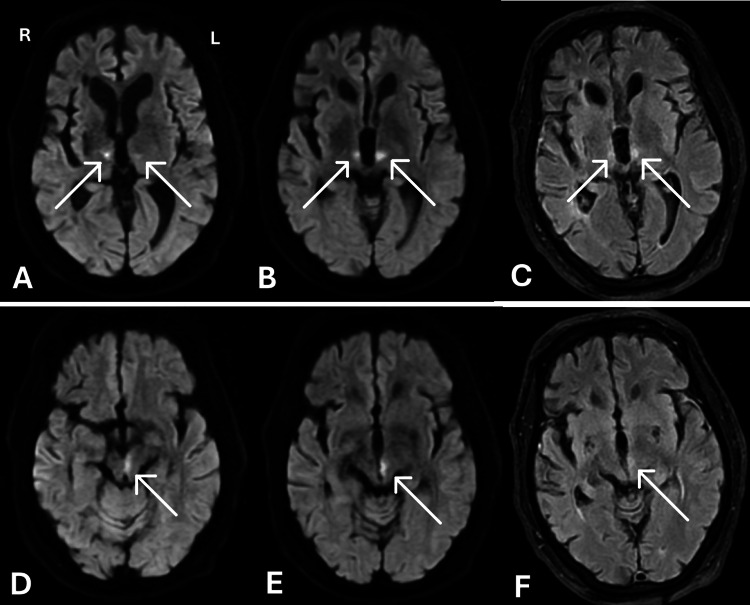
MRI performed on hospital day 1 Axial DWI (A-E) and FLAIR (C, F) images at the level of the thalamus (A-C) and midbrain (D-F). At the level of the thalamus, the arrows point to restricted diffusion involving the bilateral medial thalami, left greater than right, on DWI (A, B) and corresponding FLAIR signal hyperintensity (C). The arrow at the level of the midbrain points to left-sided restricted diffusion extending inferiorly along the left medial midbrain on DWI (D, E) and corresponding FLAIR signal hyperintensity (F).
